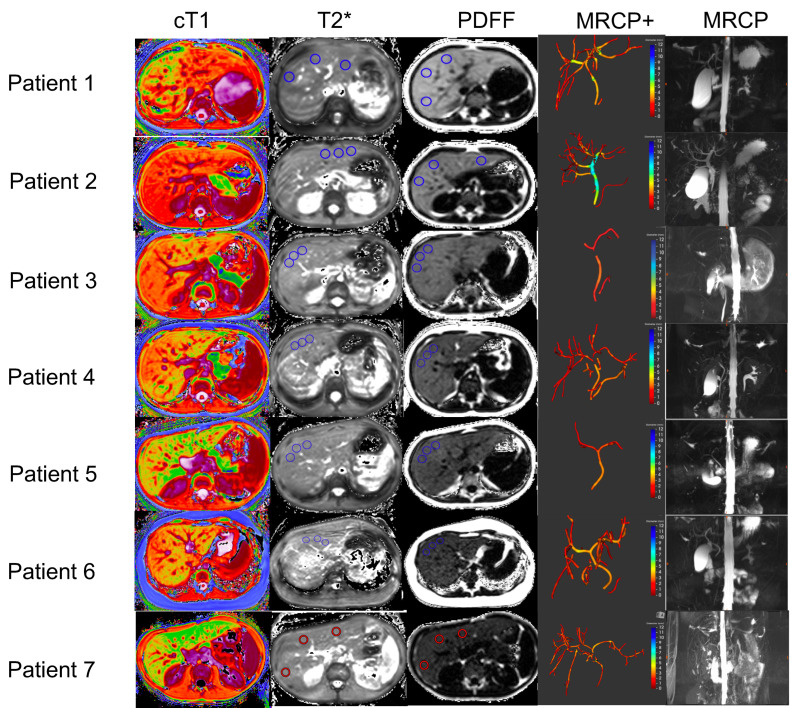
Representative biliary tree models, and cT1, T2*, and PDFF maps from all Wilson’s disease patients recruited in this study. In addition, the MRCP+ models of the biliary tree and the original MRCP images are also shown for each patient. In the cT1 maps, lower values (cooler colors) represent areas with lower cT1 values, and therefore, lower fibro−inflammation, whereas higher cT1 values (warmer colors) represent areas of the liver with higher fibro−inflammation. In the MRCP+ images, strictures are shown in red, whereas dilatations are shown in blue. cT1: corrected T1; PDFF: proton density fraction (MRI liver fat); magnetic resonance cholangiopancreatography (MRCP); quantitative magnetic resonance cholangiopancreatography (MRCP+).
